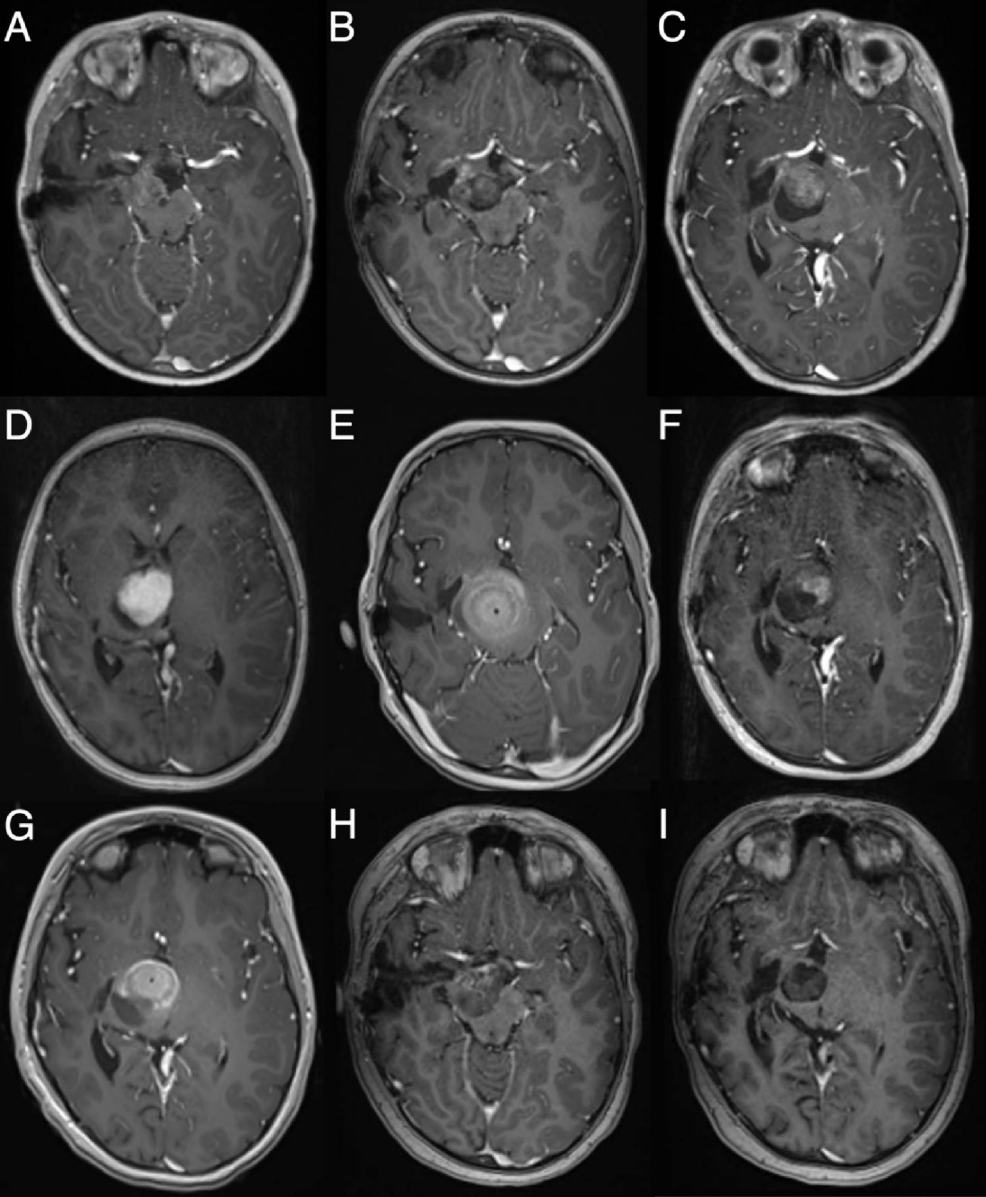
Serial T1-contrast-enhanced axial MR images of case #2. A After the resection showing minimal residual tumor, that gradually expanded on follow-up (B, C). C At the first consideration of LITT, D continued growth before the first LITT, lesion is > 2 cm in diameter and requires more than one trajectory. E At the end of the first ablation. F After 6 months, before the second ablation. G At the end of the 2nd LITT procedure. H, I At 2- and 3-year follow-up, respectively
